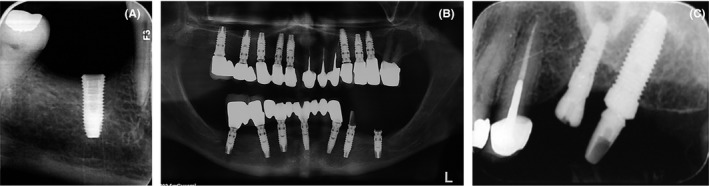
A, B and C; Broken implant abutments observable on radiographs
